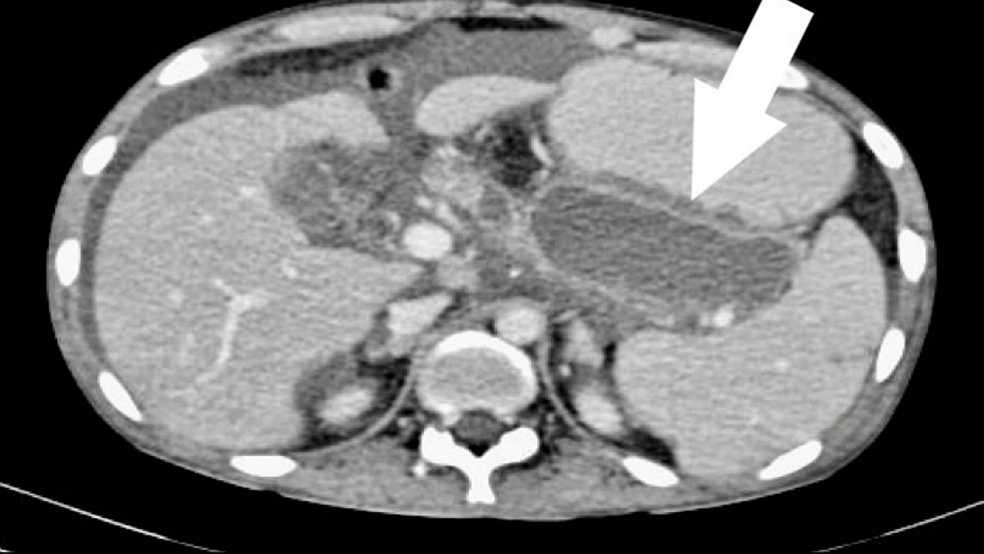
Contrast-enhanced CT axial section showing a well-defined cystic lesion around the tail of the pancreas with a thin enhancing wall.
